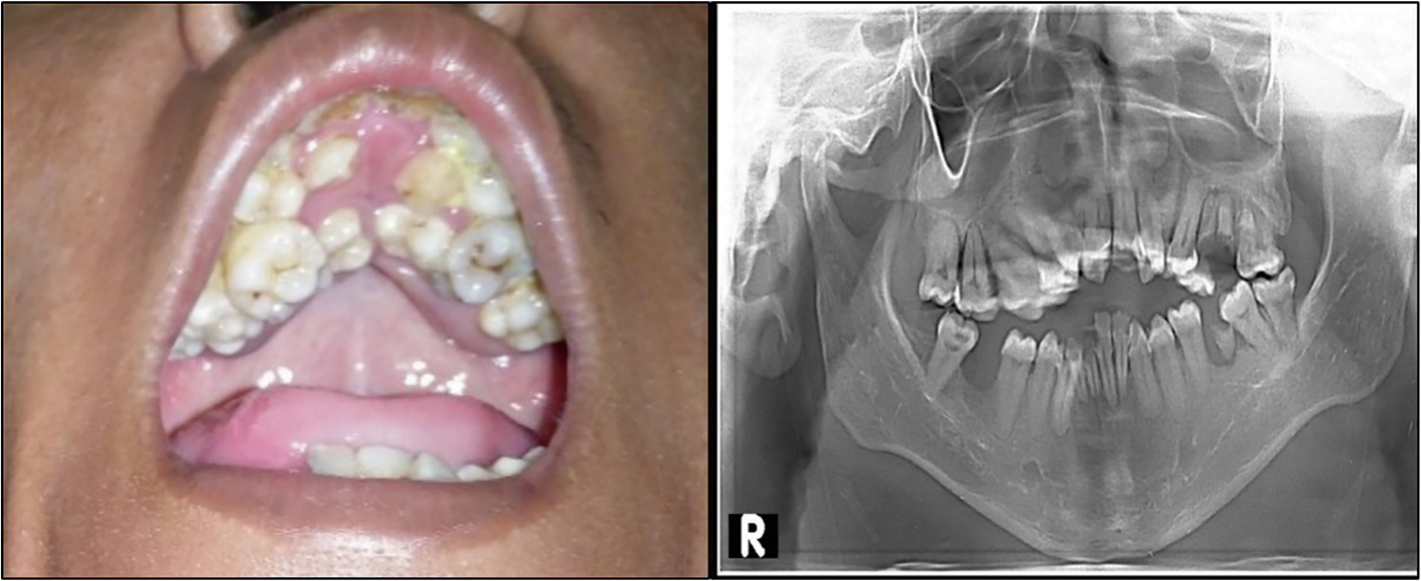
Intraoral appearance of the patient. Photograph showing V-shaped maxillary arch, crowding of teeth, prominent jaw, and high arch palate. Panoramic X-ray showing hypoplastic and retruded maxilla
